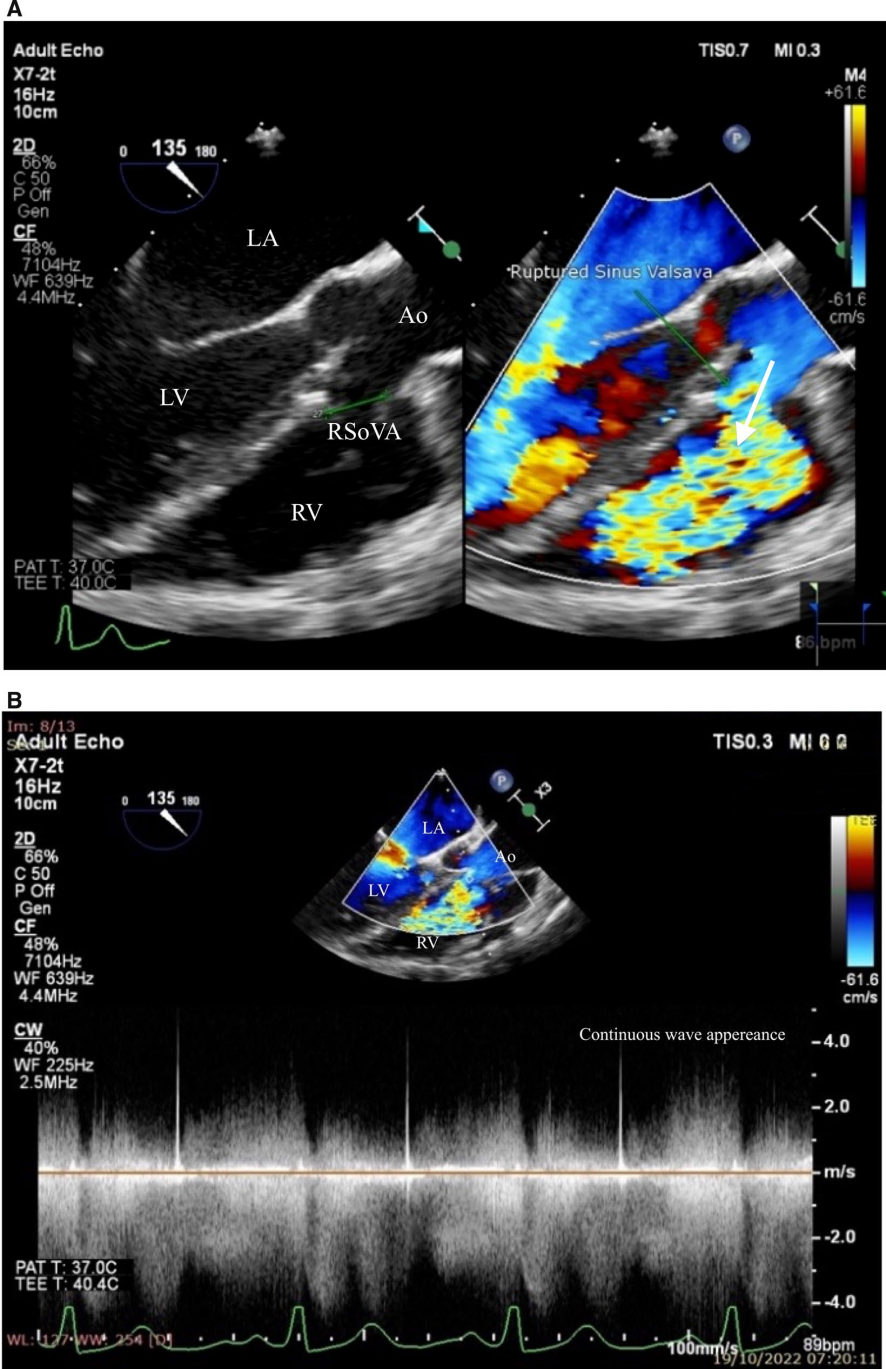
Transoesophageal echocardiography showed A a rupture of the Valsalva aneurysm sinus with multiple end- hole with flow heading towards the RV, coming from the aorta to RV (arrow). B Continuous wave appearance can be seen CW doppler was done. Ao = Aorta; RV = Right ventricle; LV = left ventricle; LA = left atrium; RSoVA = ruptured sinus of Valsalva aneurysm
